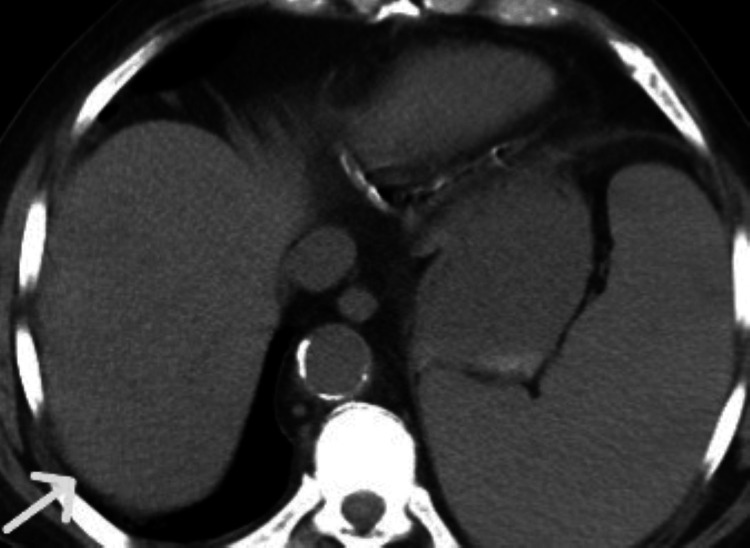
CT scan with enlarged spleen on the left CT: computed tomography
